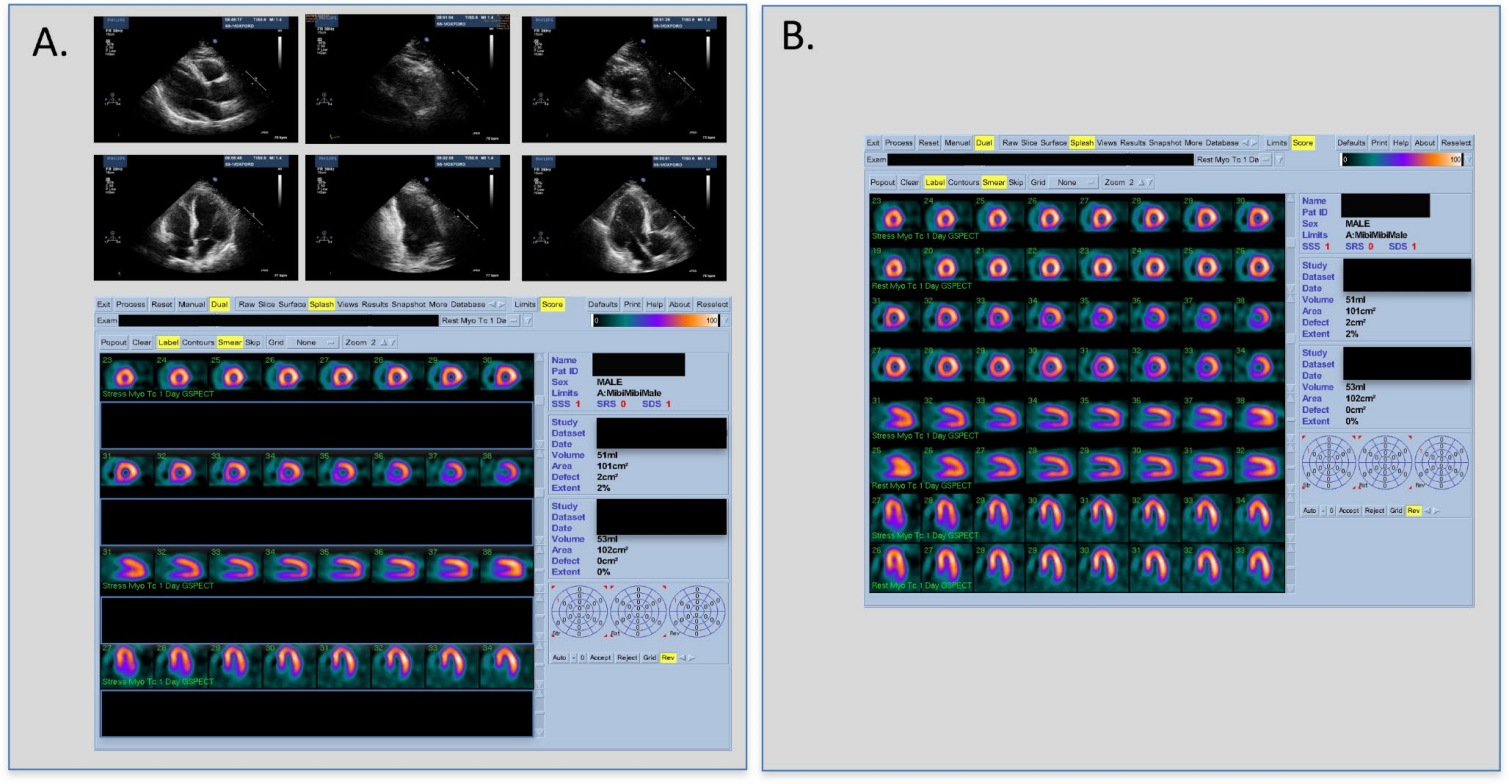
A dual modality cardiac strategy with echocardiography-nuclear assessment (ECHO-MPS) imaging and routine MPS in a patient. (Panel A - example of ECHO-MPS set of TTE images and stress SPECT images. Panel B – routine MPS with stress and rest SPECT images. Abbreviations: MPS, myocardial perfusion scintigraphy; SPECT single photon emission computed tomography; TTE, transthoracic echocardiogram
